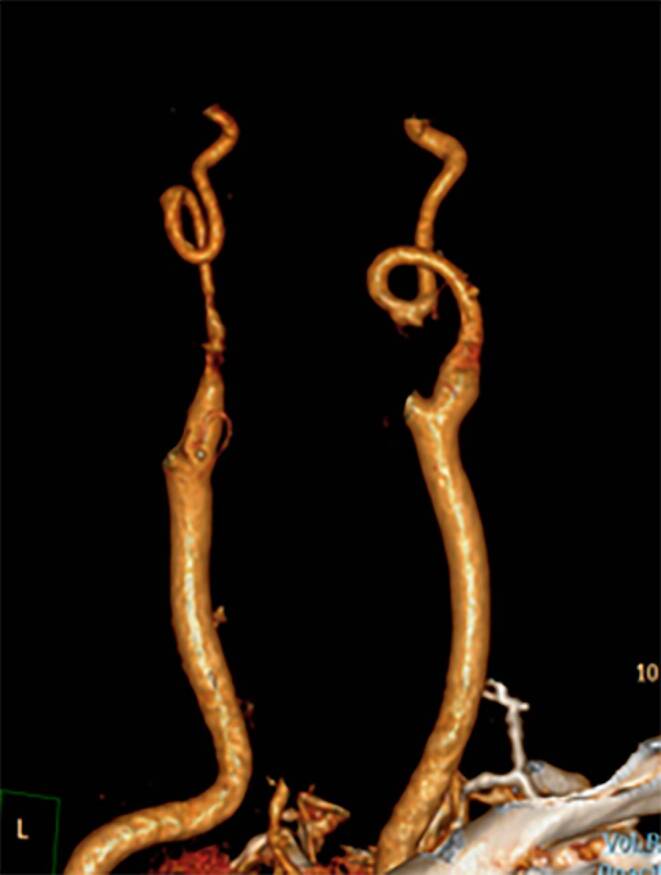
3D reconstructed CT image demonstrating coiling of both internal carotid arteries in a circular configuration
